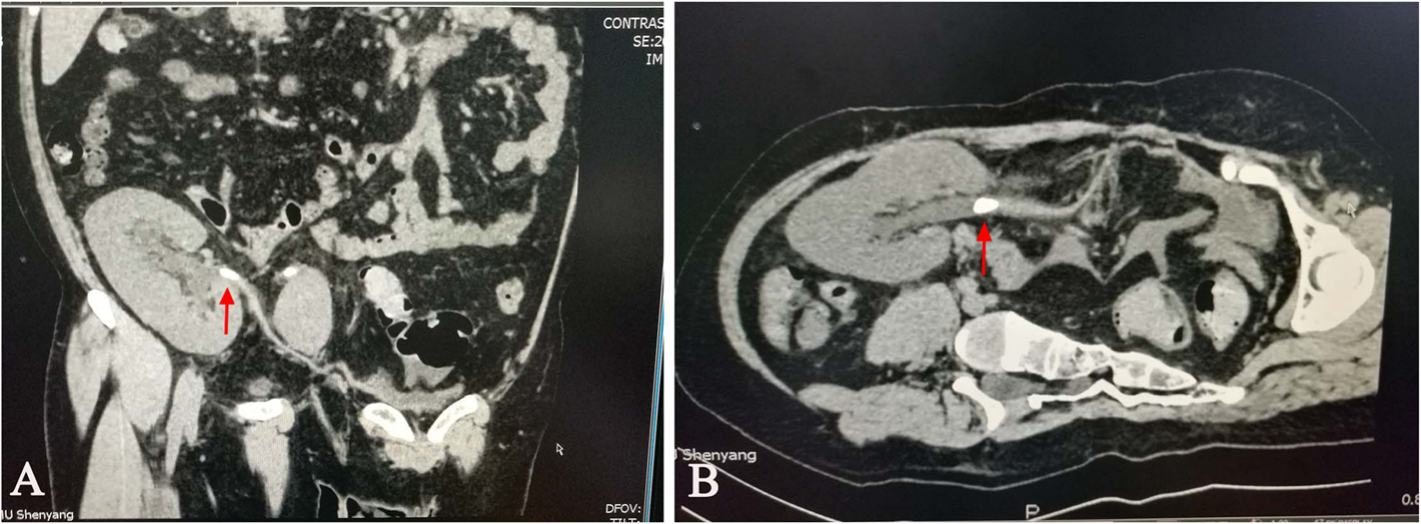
Preoperative CT scan showing a ureteral calculus in the ureteropelvic junction. a Coronal plane, b transverse plane
